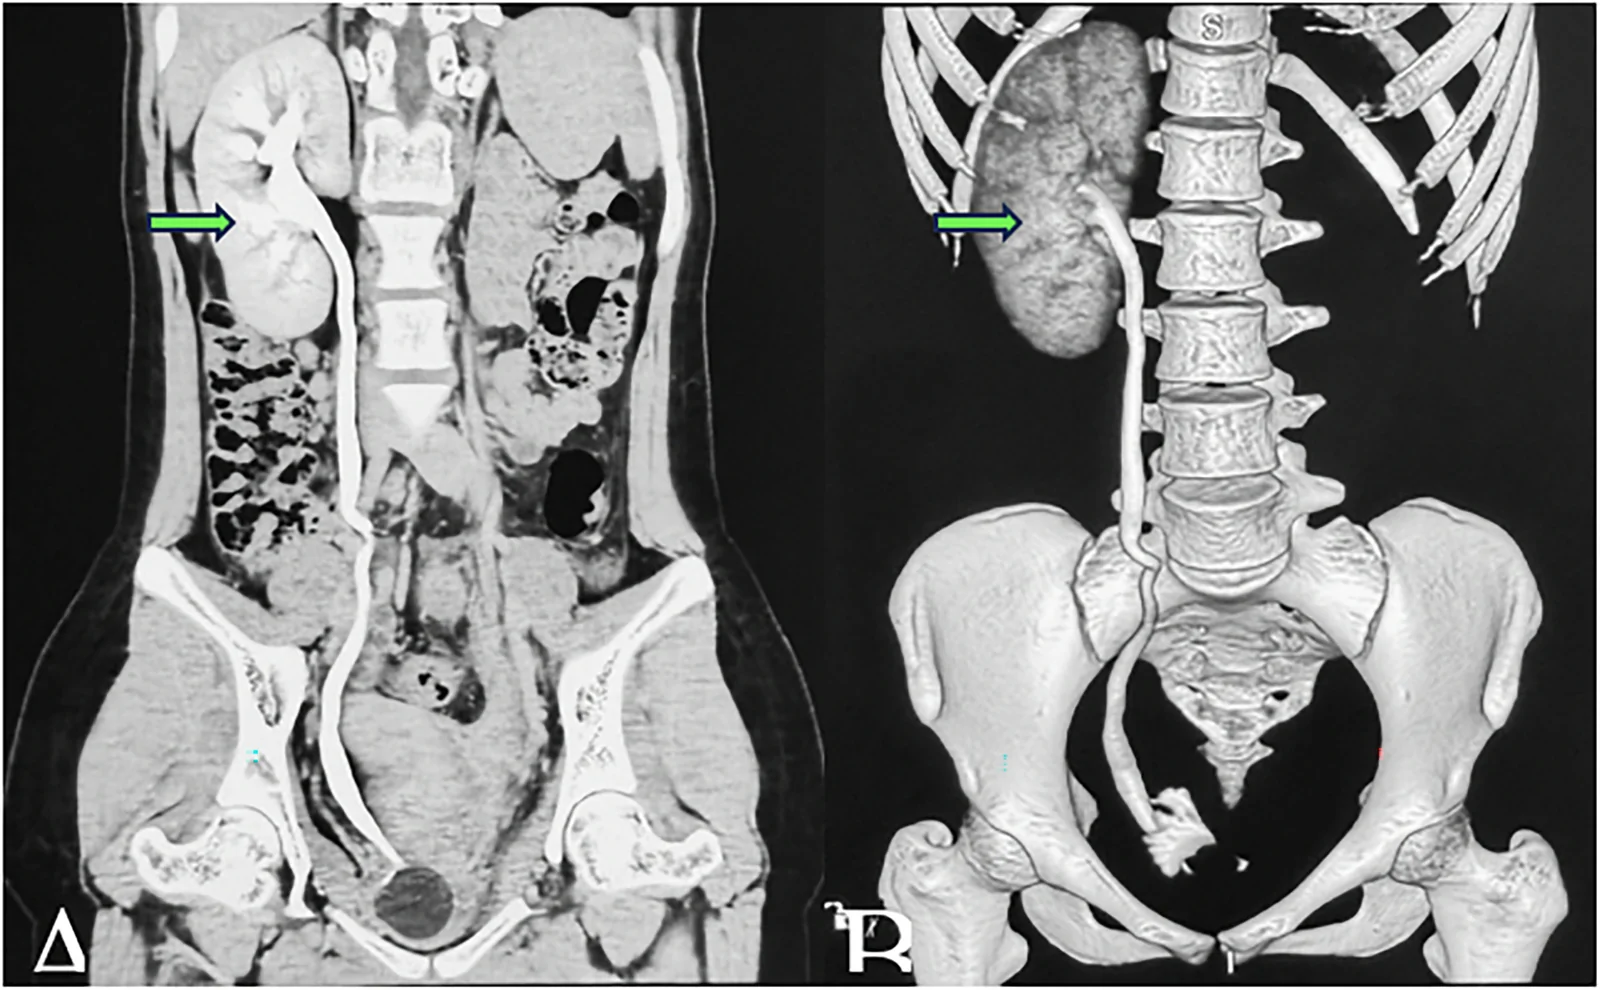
CT urography demonstrating the associated urinary tract anomaly. (A) Coronal contrast-enhanced CT urography (excretory phase) demonstrates complete absence of the left kidney and left ureter, consistent with left renal agenesis. The right kidney (marked with a green arrow) is normal in size and morphology, with homogeneous contrast enhancement and preserved excretory function, evidenced by opacification of the right renal collecting system and ureter. (B) Three-dimensional volume-rendered CT urography reconstruction confirms complete absence of the left kidney and ureter. The right kidney (marked with a green arrow) and ureter are normally developed, with preserved continuity of the right urinary tract extending to the urinary bladder.
